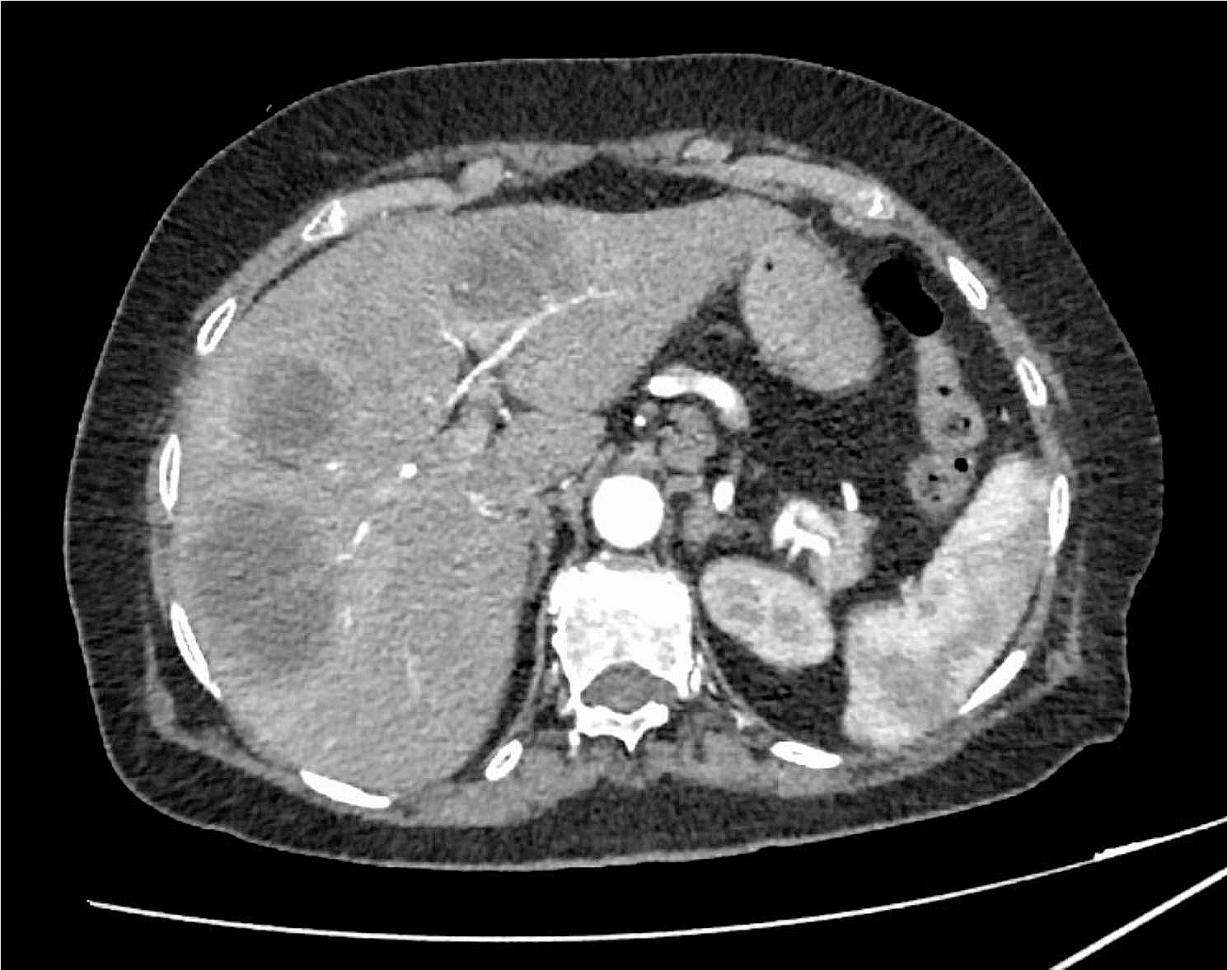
Contrast-enhanced abdominal CT showing the presence, in the liver, of three roundish lesions (SIII-SIV, SV and SV-SVIII) with a cystic morphology. They appear as peripherally enhancing e centrally hypoattenuating lesions; we can also see they are surrounded by an inner ring and they are provided of multiple septa. Due to the peculiar pattern of enhancement, these lesions were considered to be consistent primarily with pyogenic liver abscesses
